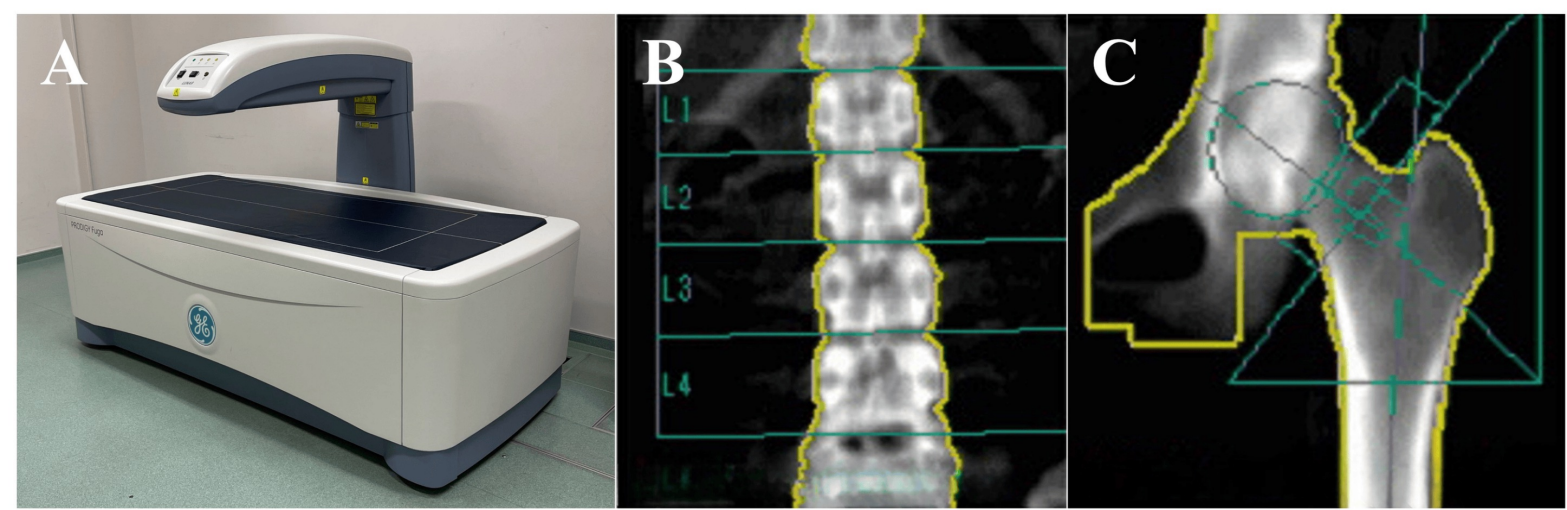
DXA and BMD analysis Dual-energy X-ray absorptiometry (DXA) (A) was used to measure lumbar spine bone mineral density (LSBMD) (B) and femoral neck bone mineral density (FNBMD) (C).
